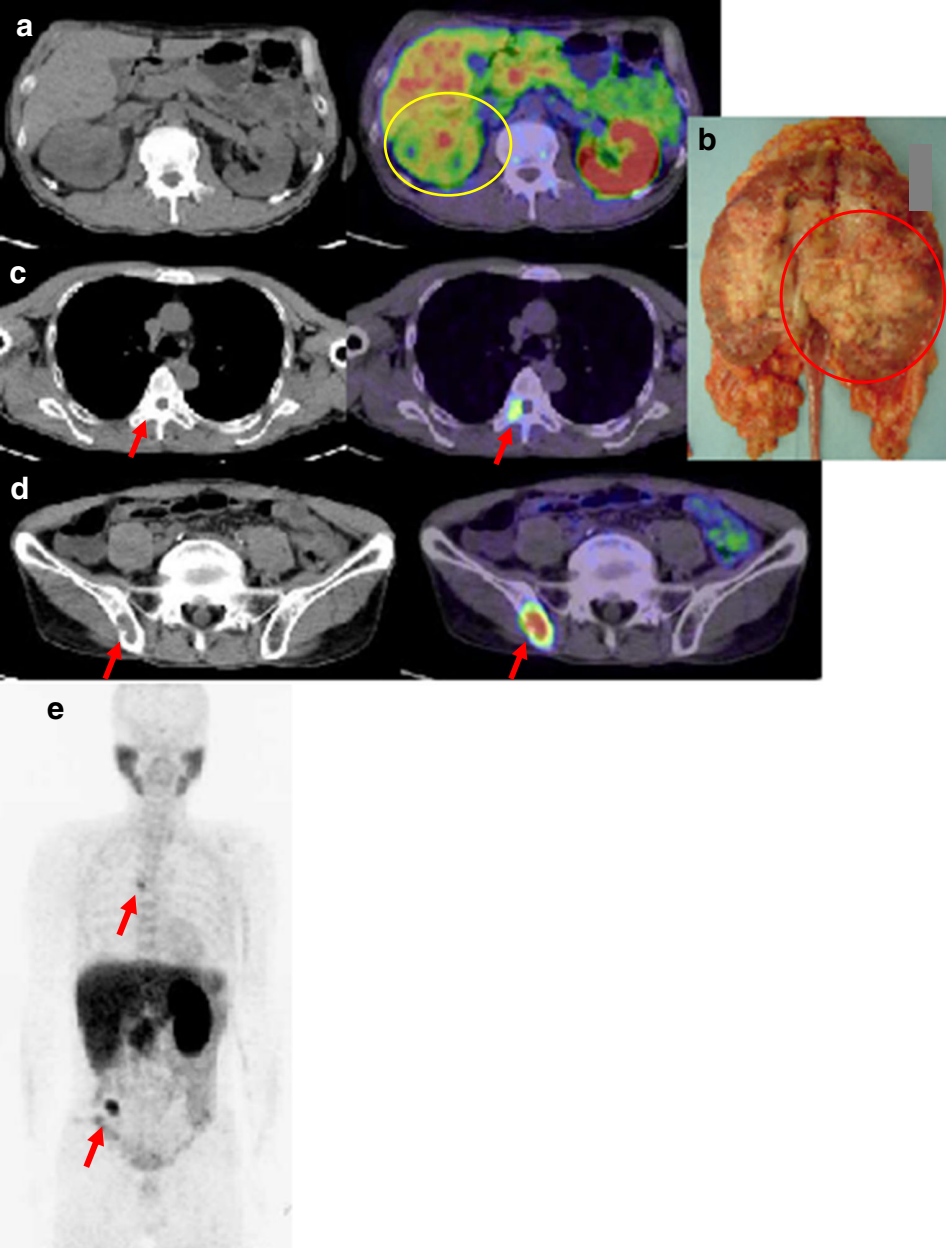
Urothelial carcinoma of the right renal pelvis in patient 12. a Choline PET/CT images of the primary tumour at 10 min after injection (SUVmax 7.85). b Photograph of the primary tumour after resection. *Circles* tumour sites. c, d Choline PET/CT images of the metastatic lesions at the 12th thoracic spine (SUVmax 7.36, c) and the right iliac bone (SUVmax 10.39, d) at 10 min after injection. *Arrows* bone metastases. e Maximum intensity projection image. The metastatic lesions in the sixth thoracic spine and the right iliac bone are seen (*arrows*)
